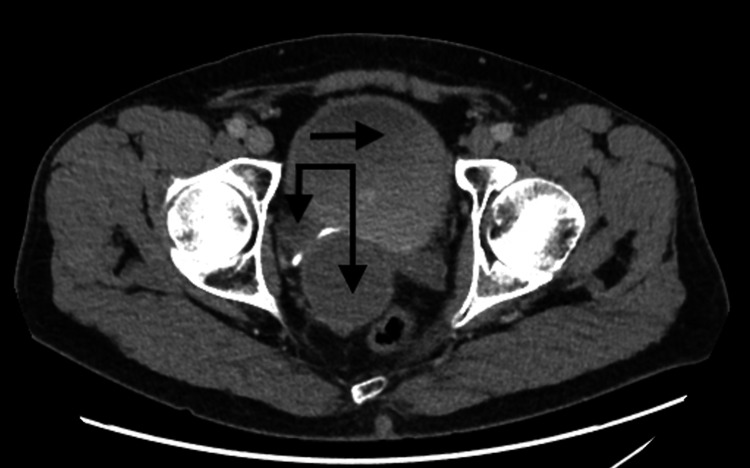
Transverse view of CT abdomen and pelvis with contrast showing bladder diverticulum (vertical arrows) noted wrapped around the distal ureter as it enters the bladder, marked with a horizontal arrow
